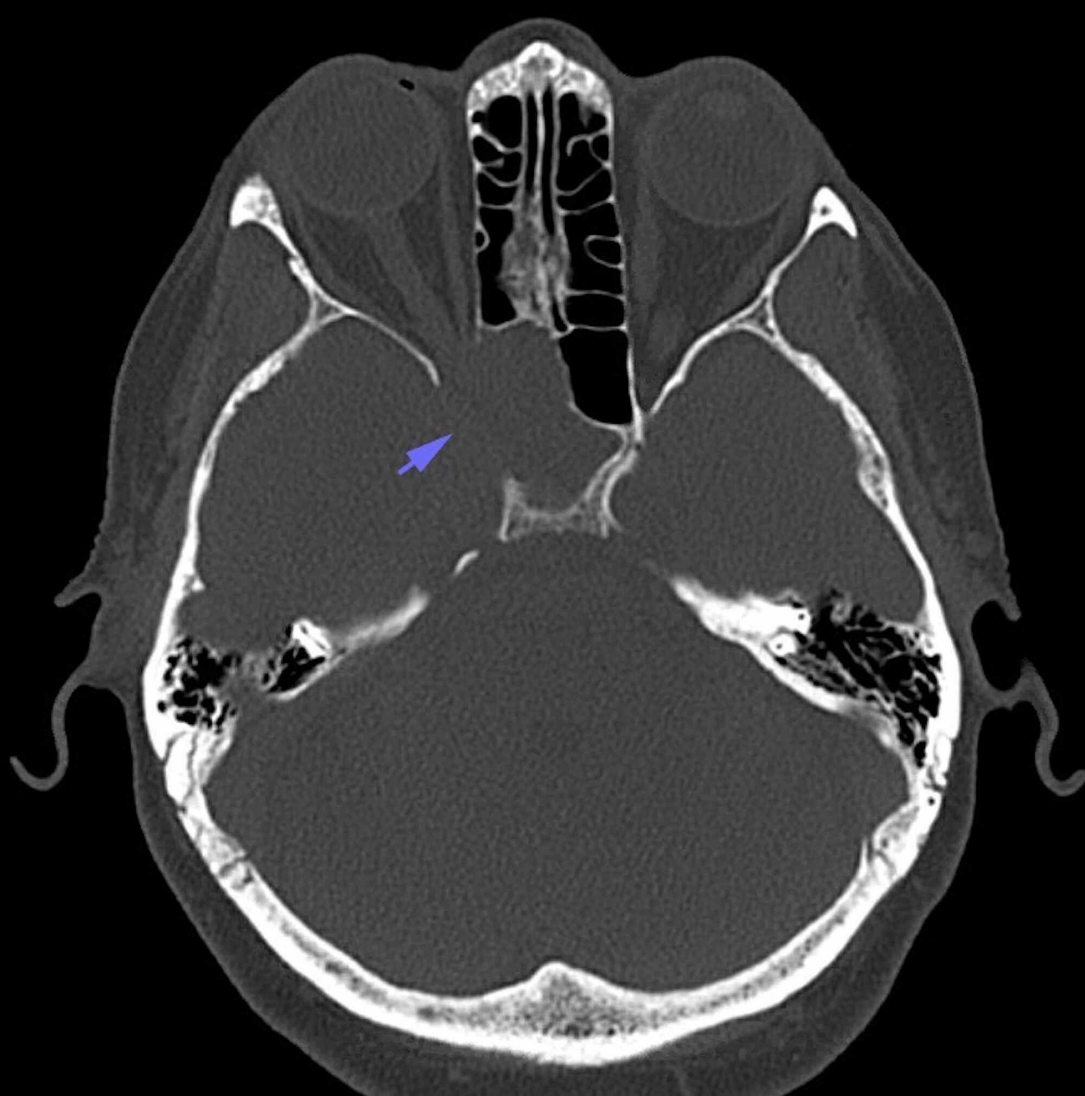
Axial brain CT showing osseous erosion and remodeling by a right-sided plasmacytoma (arrow). CT, computed tomography
